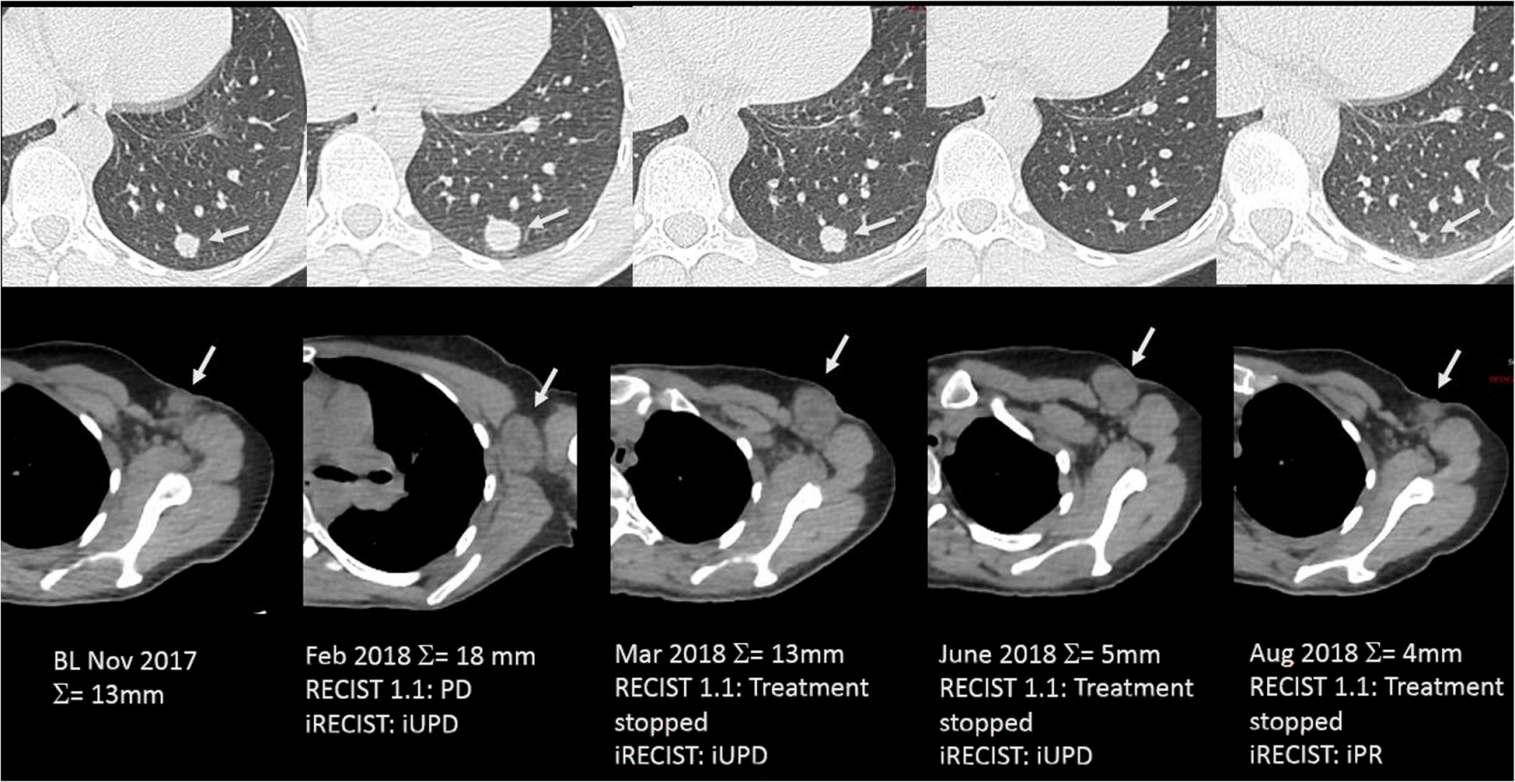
Comparison of RECIST 1.1 and iRECIST criteria for evaluation of a 45-year-old woman with metastatic melanoma treated with ipilimumab (antiCTLA-4) and nivolumab (anti-PD-1). Baseline CT image (November 2017) shows a 13-mm lung metastasis (target lesion, upper panel, arrow) and a 10-mm short-axis axillary lymph node (non-target lesion, lower panel, arrow). On a 3-month follow-up, both lesions enlarged with an increase of 38% of the target lesion leading to a progressive disease (PD) and a stop of treatment according to RECIST 1.1 and an unconfirmed PD with maintained treatment according to iRECIST criteria. On the two following CT examinations (March and June 2018), the lung metastasis decreased in size, but the axillary lymph node was stable (unconfirmed PD) but still significantly enlarged compared to the baseline (still unconfirmed PD according to iRECIST criteria). Finally, on August 2018, CT images showed a decrease in size of both lesions, confirming the pseudoprogression with a response assessed to be -70%, leading to a partial response according to iRECIST criteria
